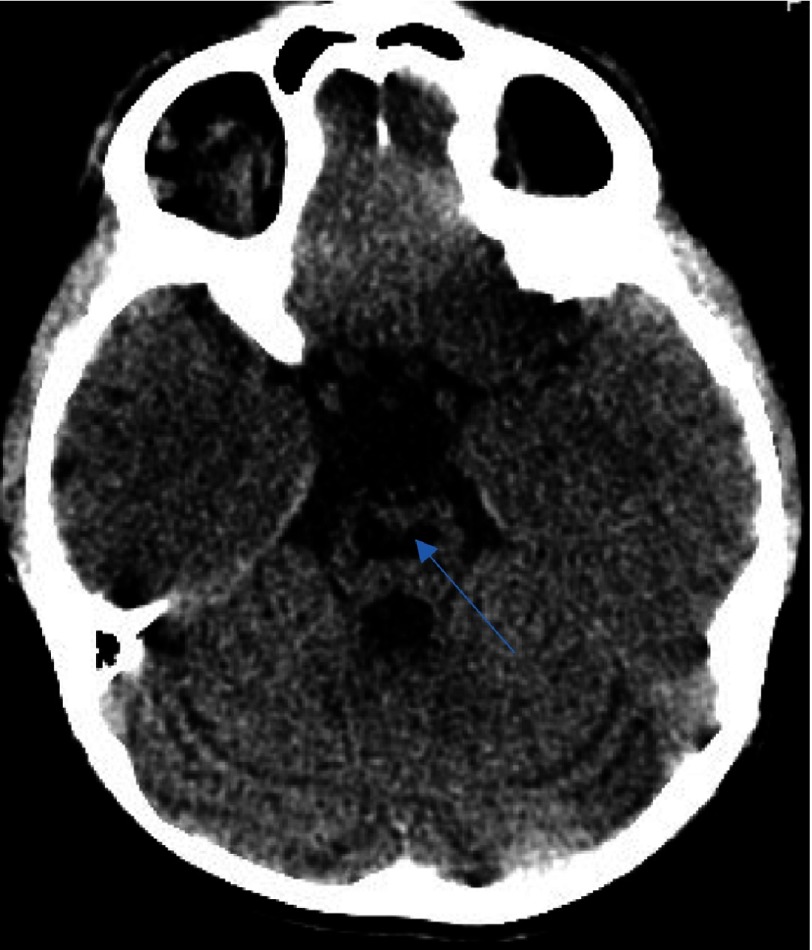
Axial CT scan at even higher level, verifying extension of the infarction into the mesencephalon (arrow).
